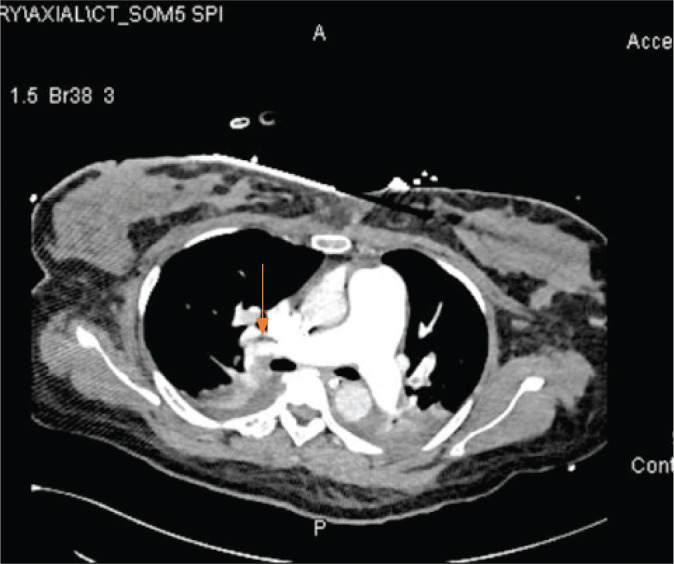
CT chest showing pulmonary embolism.
